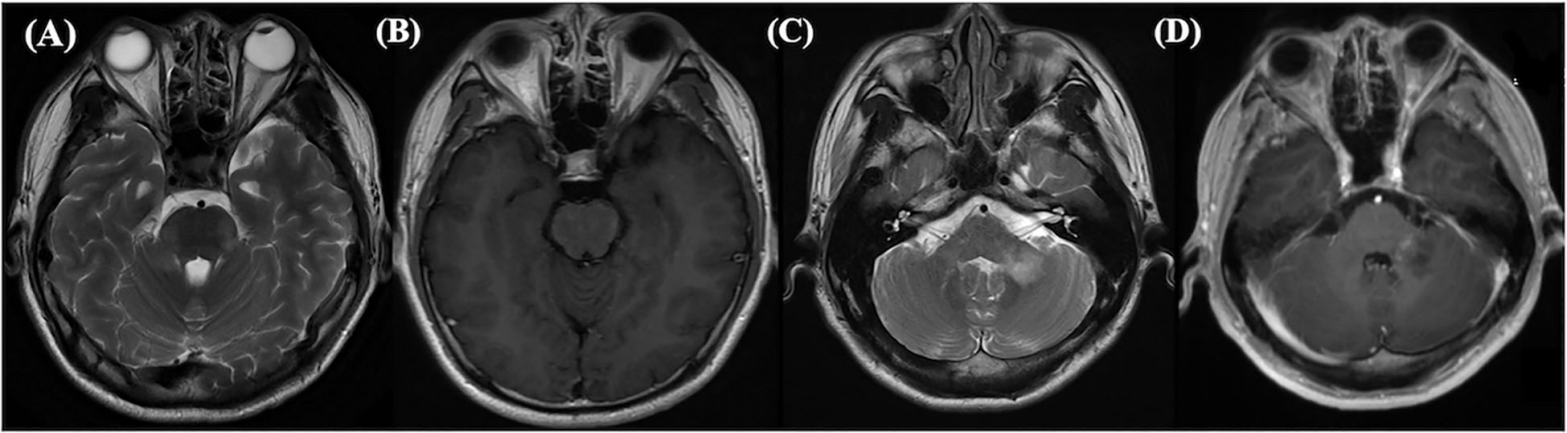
This abnormal MRI was from a 28-year-old male suffered from NMDARE then transferred to MOGAD. Axial T2-weighted (A) and enhancement (B) images showed hyperintense signals in brain pons, bilateral middle cerebellar peduncle and medulla oblongata at the first relapse. At the second relapse, left cerebellopontine area showed hypersignals in T2-weighted (C) and enhancement (D). Both relapses were double antibody positive.
